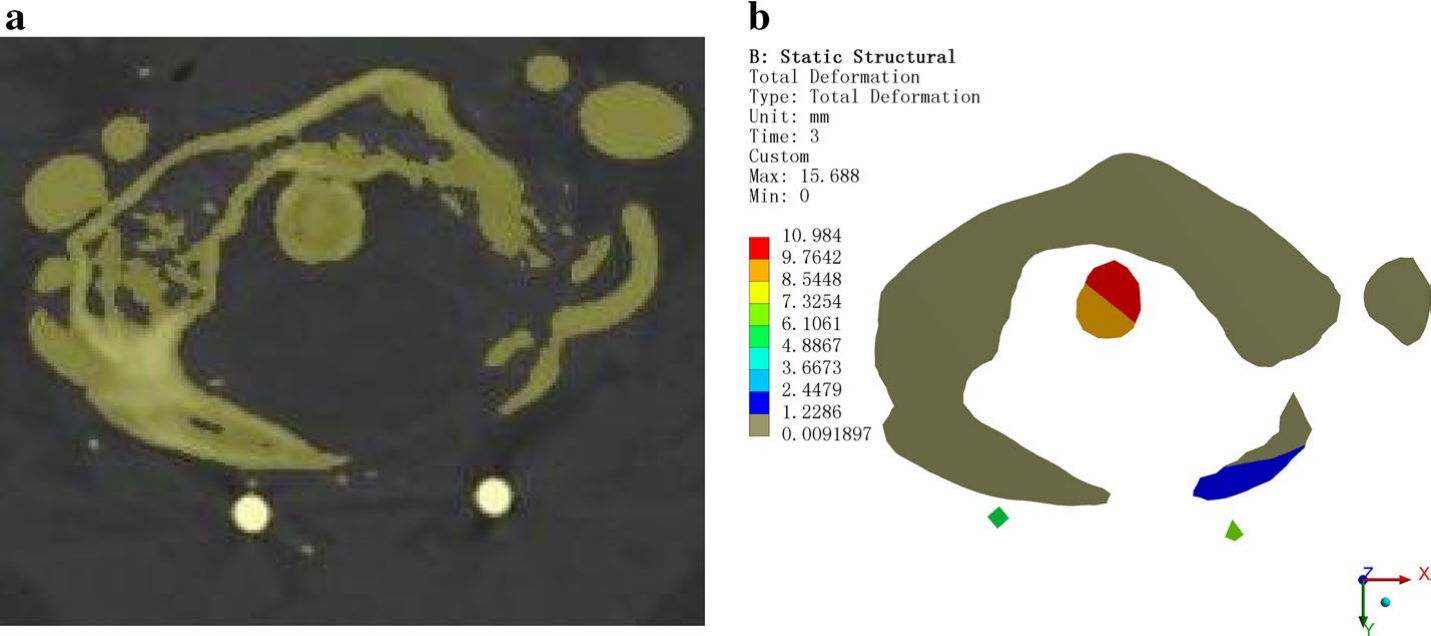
Comparison chart for the horizontal plane. a CT. b ANSYS displacement cloud chart
